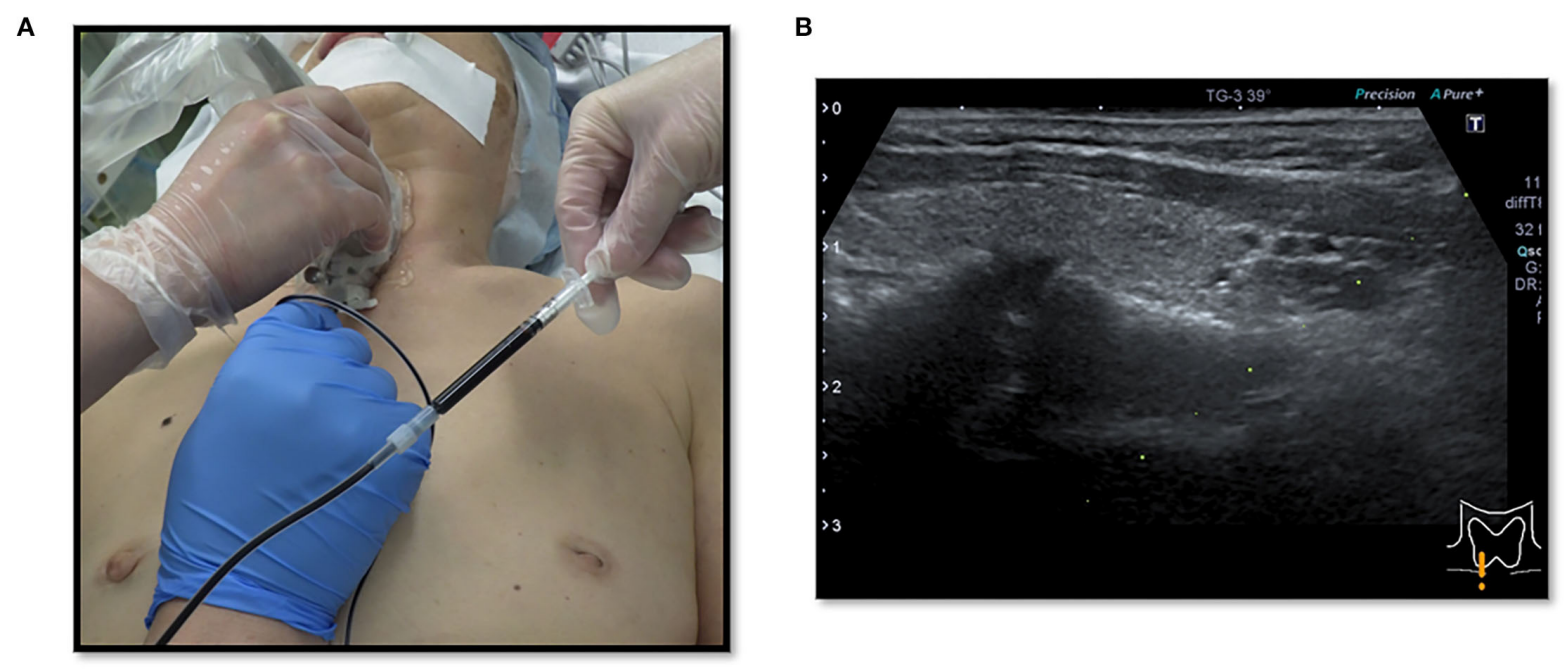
(A,B) Cervical neck ultrasound-guided injection of indigo carmine for marking EPA (dye method).
